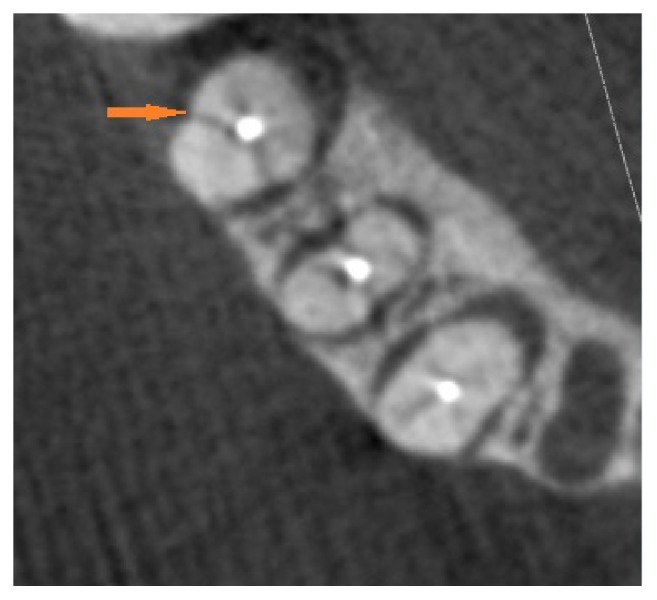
CBCT, axial view; red arrow: VRF in gutta-percha filled canals.
